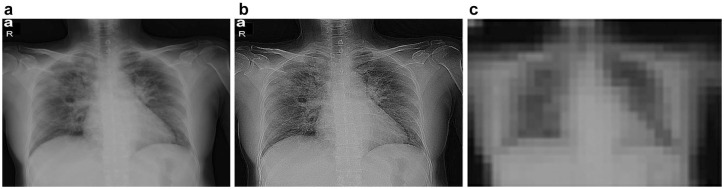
Pre-process of X-ray images. (a) Original chest X-ray image, (b) sharpened image using a Laplacian filter, and (c) average pixel per node (APPN)-applied image (10× enlarged).
